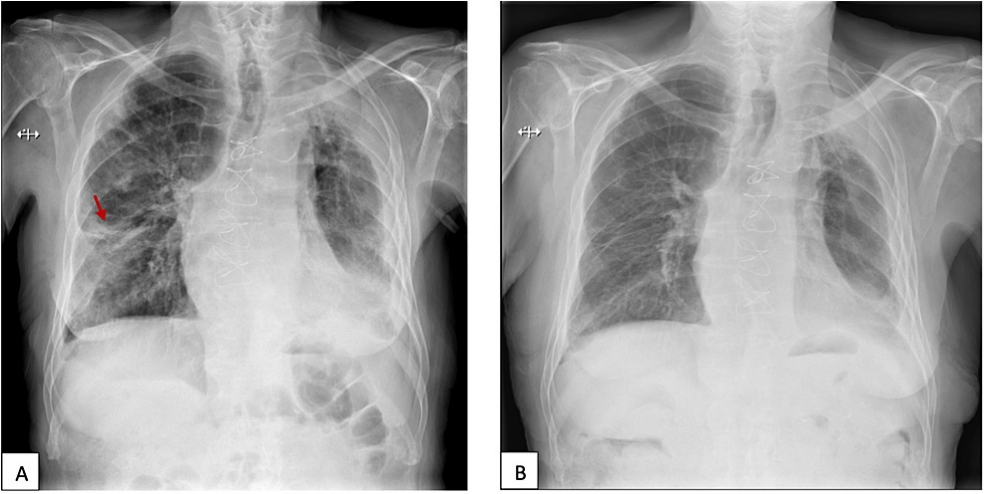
Chest radiographs of Patient 1 on admission (A) and one month after corticosteroids (B) It shows regression of the lung infiltrate on the right after a month of treatment with corticosteroids (red arrow) and stable pleural effusion on the left.
